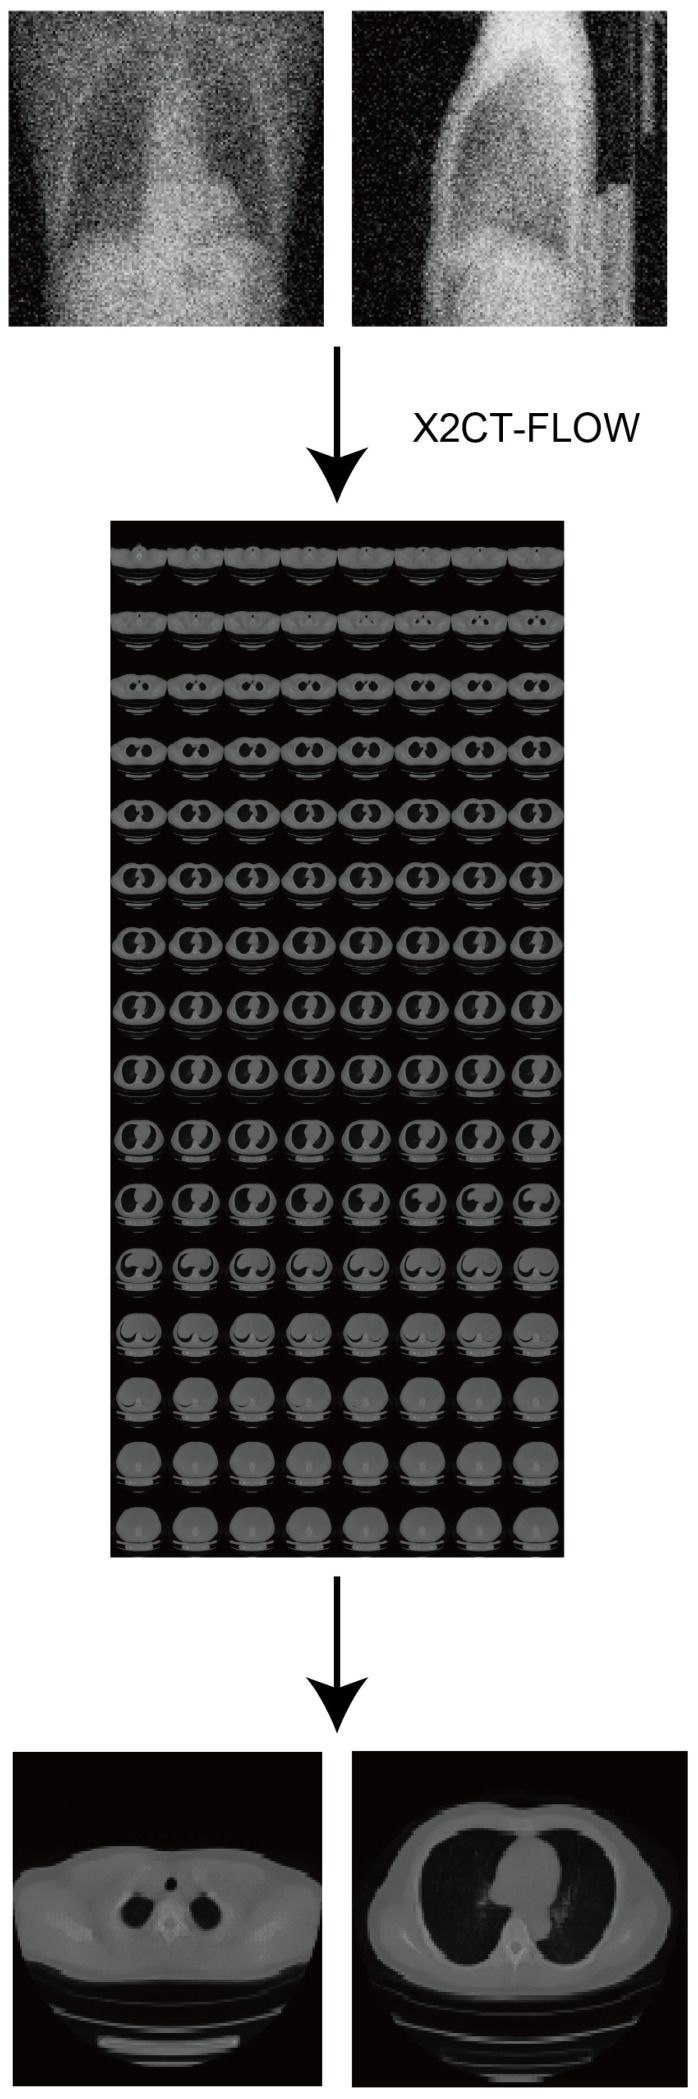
X2CT-FLOW can find the optimum 3D chest CT image (the middle and bottom) from a single or a few noisy projection images (the top) with MAP reconstruction. Scales of the images are not the same.
